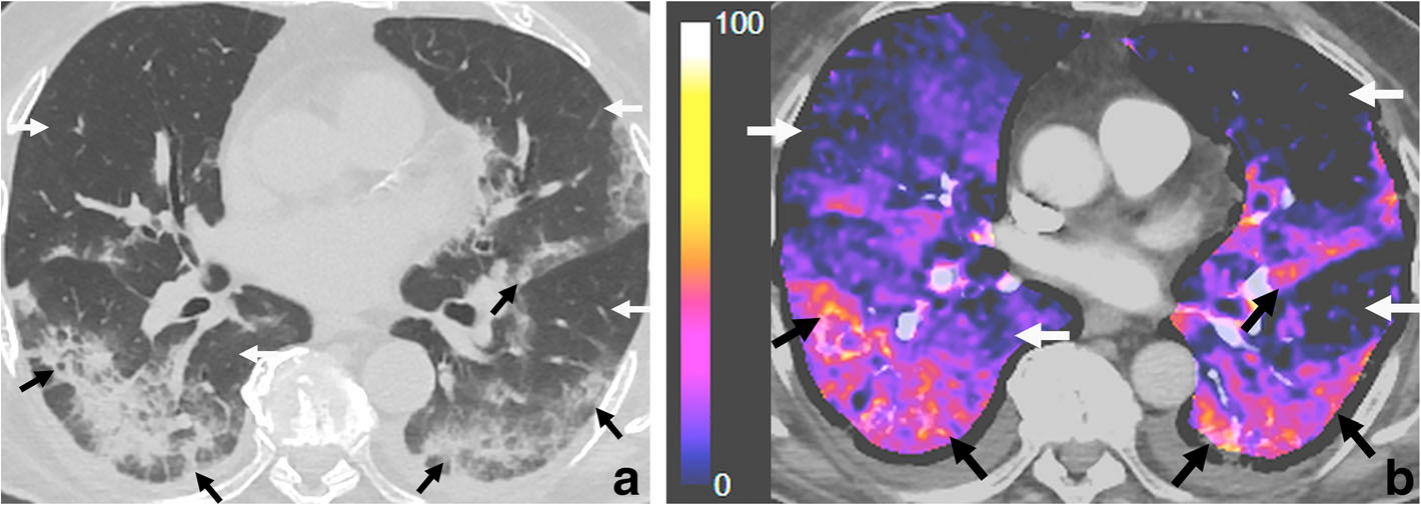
a, b Prominent hypoperfusion in the well-aerated lung and hyperperfusion in areas of injured lung. Seventy-eight-year-old male patient, RT-PCR-confirmed COVID-19, 10 days since symptom onset, with hypoxemia, (PaO_2_/FiO_2_) 206, d-dimer 1600 ng/mL progressively increasing. There are extensive foci of consolidation and ground-glass opacities, associated with septal thickening, with a predominantly posterior and subpleural bilateral distribution, which correlate with the areas of hyperemia and iodine pooling in subtraction CT iodine maps (black arrows). There are areas of markedly decreased perfusion in both lungs, which correlate with the apparently healthy lung parenchyma in conventional chest CT images (white arrows). Bilateral pleural effusion. This could be explained by an increased blockage of ACE2 receptors in the lung endothelium, leading to increased local levels of angiotensin II, which leads to vasoconstriction and ventilation/perfusion mismatch. This patient was managed with invasive mechanical ventilation, with highly compliant lung parenchyma, in accordance with the type 1 or L phenotype described by Gattinoni et al.
